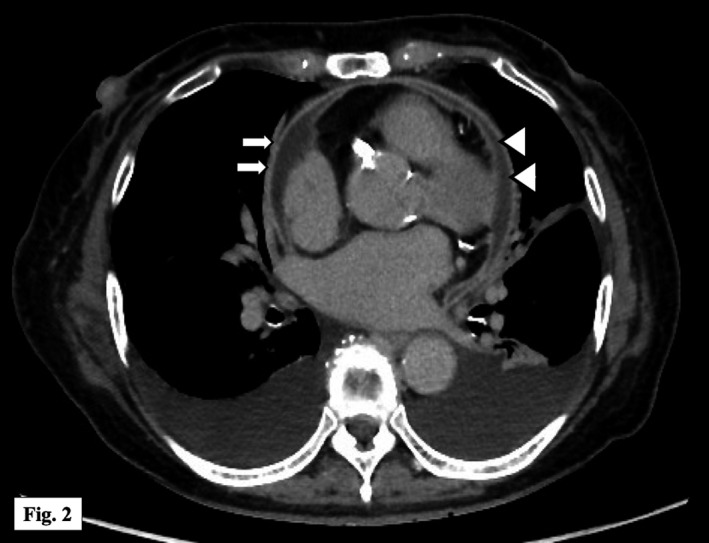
Contrast chest CT on day 10 demonstrated an enlargement of the pericardial effusion (white arrowhead) and thickened pericardium (white arrow)
